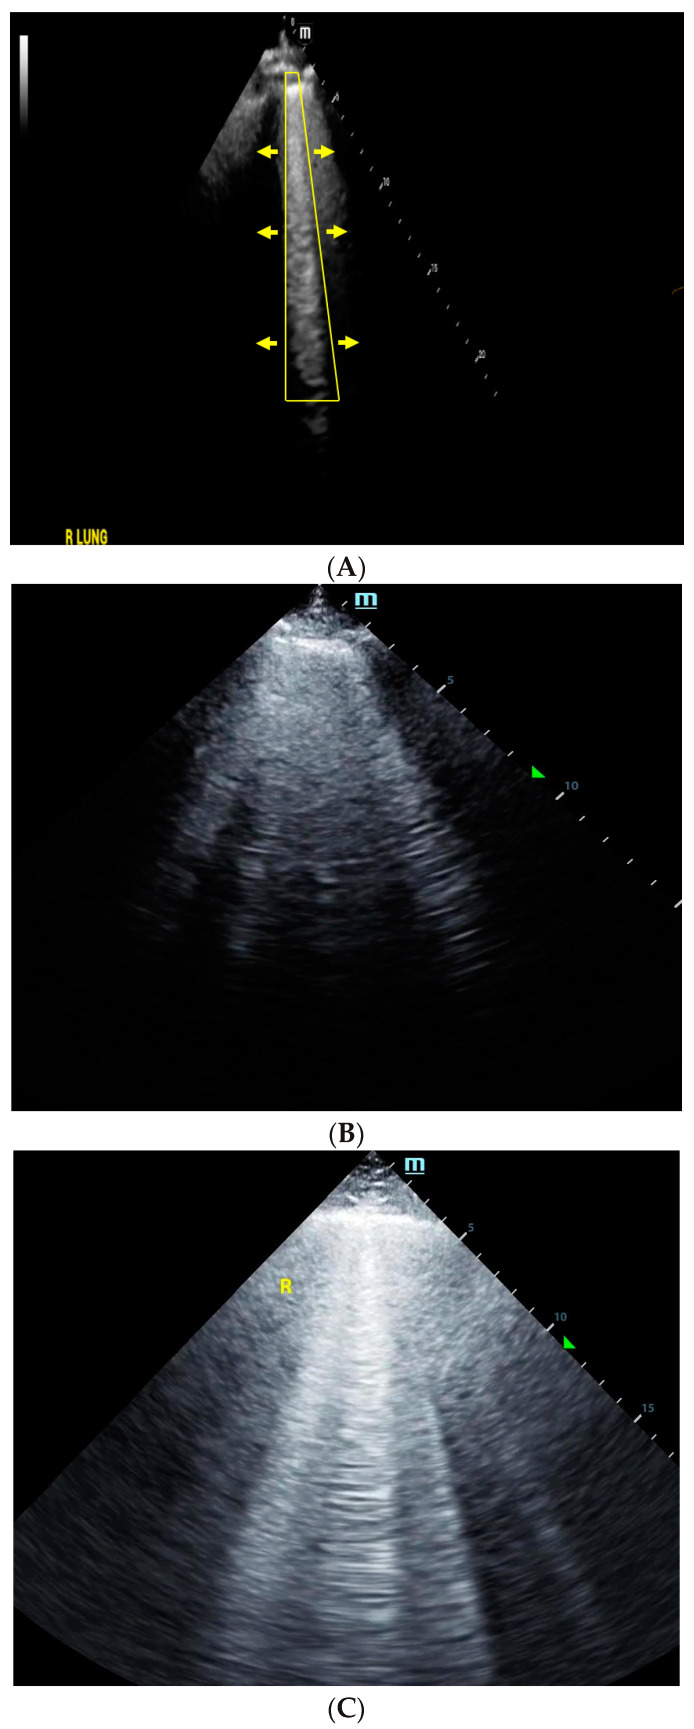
(A) Abnormal lung ultrasound, with B-line pattern reflecting possible interstitial edema. (B) Abnormal lung ultrasound, with B-line pattern indicating moderate interstitial edema. (C) Abnormal lung ultrasound, with B-line pattern indicating severe interstitial edema.
